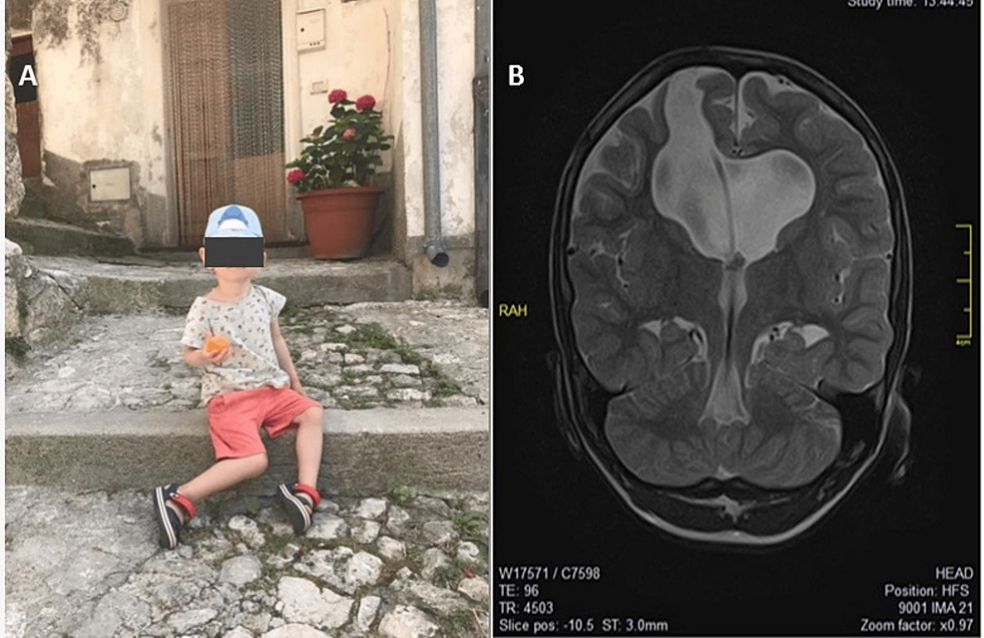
Control studies images showing the efficacy of surgical management. A - The child is having a normal life after all; B - MRI coronal cut T2 window showing the trajectory of the catheter and how it is perfectly working.
